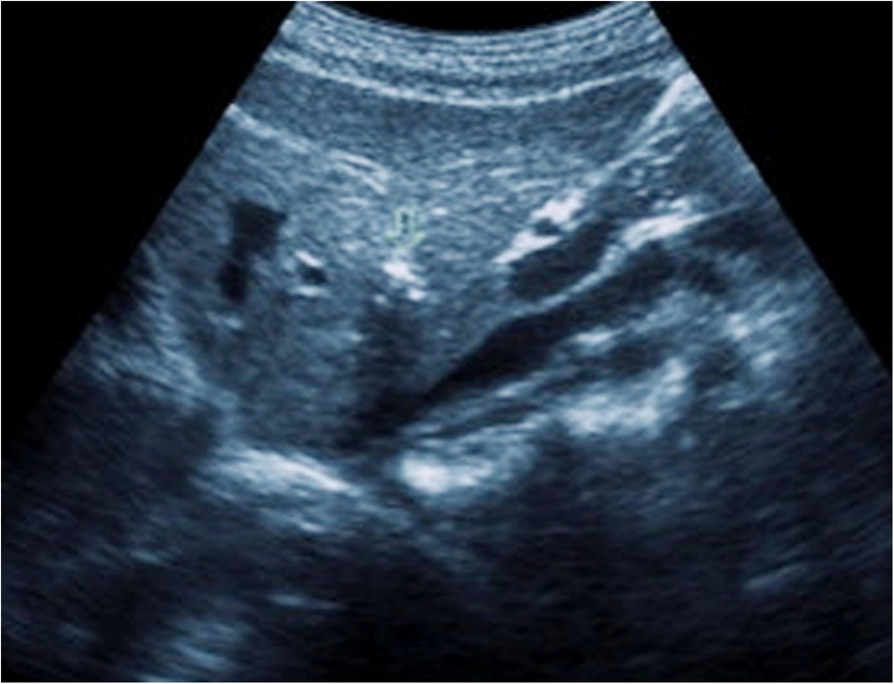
Ultrasonography (US) revealed calculus of intrahepatic duct.
